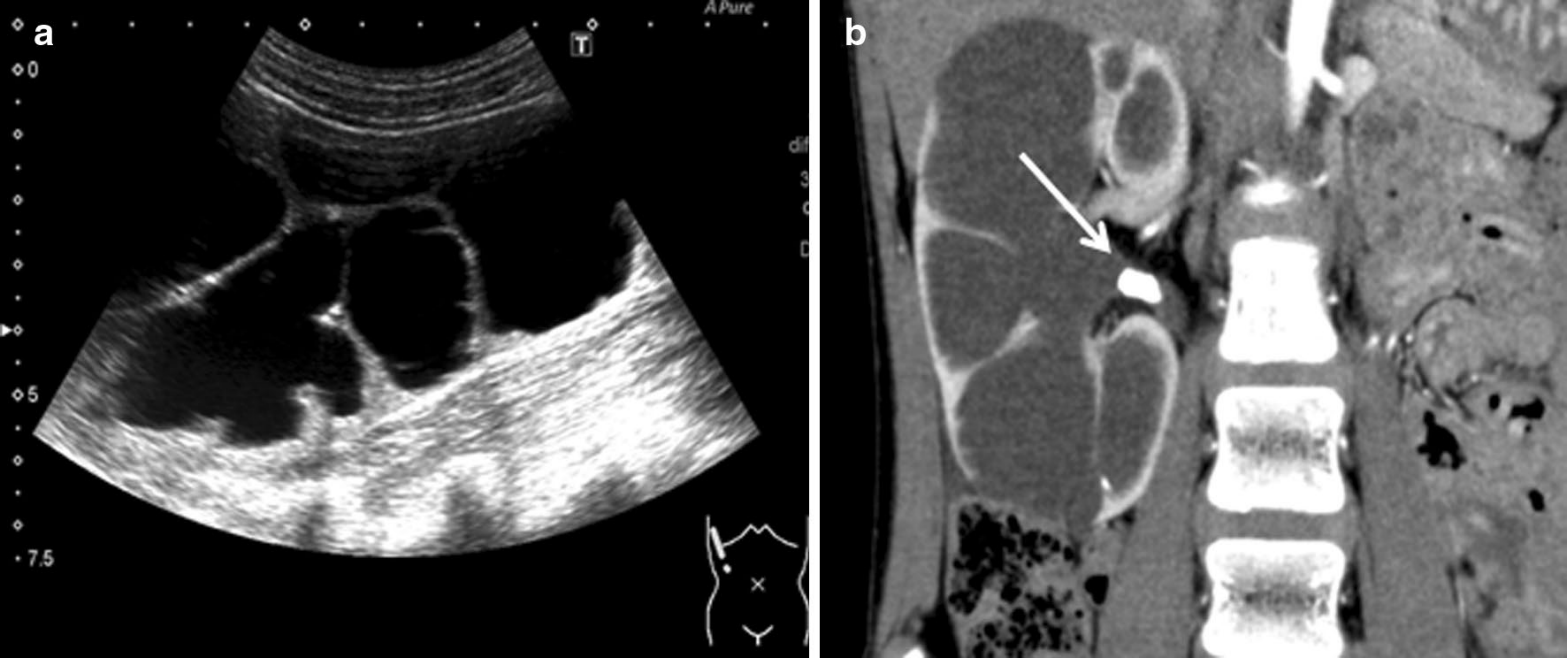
a Preoperative ultrasonography showed severe hydronephrosis. b CT scan revealed hydronephrosis and an 11 mm renal stone (*white arrow*) due to right ureteropelvic junction obstruction and thinning of the renal cortex
